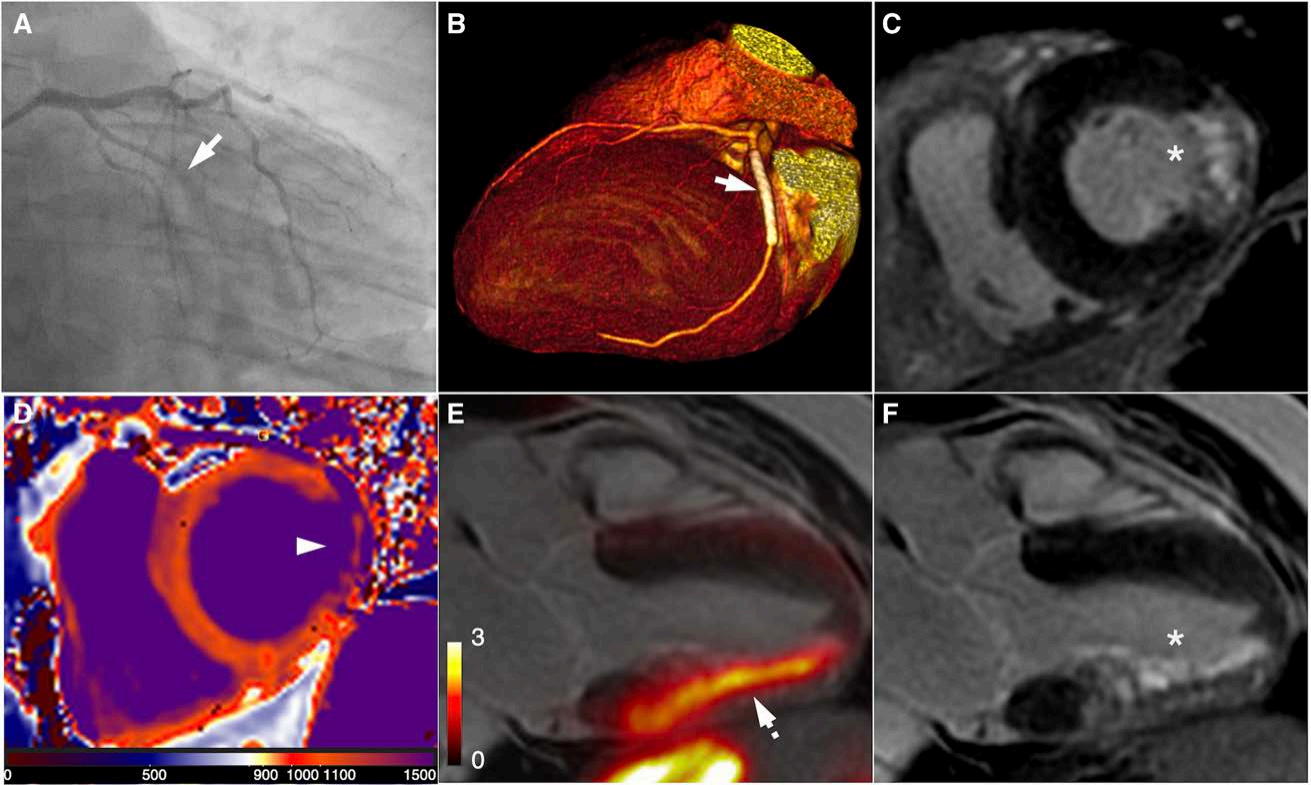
Baseline ^68^Ga-DOTATATE positron emission tomography (PET)/magnetic resonance imaging (MRI) after myocardial infarction. A, Coronary angiography showing an occluded obtuse marginal branch of the left circumflex artery (arrow) at the time of initial myocardial infarction (MI) presentation; (B) 3-dimensional volume-rendered computed tomography coronary angiography after percutaneous coronary stenting (arrow). PET/MRI performed on day 11 post-infarct: (C, short-axis view; F, 3-chamber view) late gadolinium enhancement (LGE) MRI confirms areas of near full thickness basal infero-lateral MI (asterisk) and sub-endocardial mid to apical inferolateral infarction; (D) T1-mapping in basal short-axis view corroborating the extent of infarct (arrowhead); (E) increased ^68^Ga-DOTATATE PET uptake (dashed arrow) co-localizes with the area of infarct.
